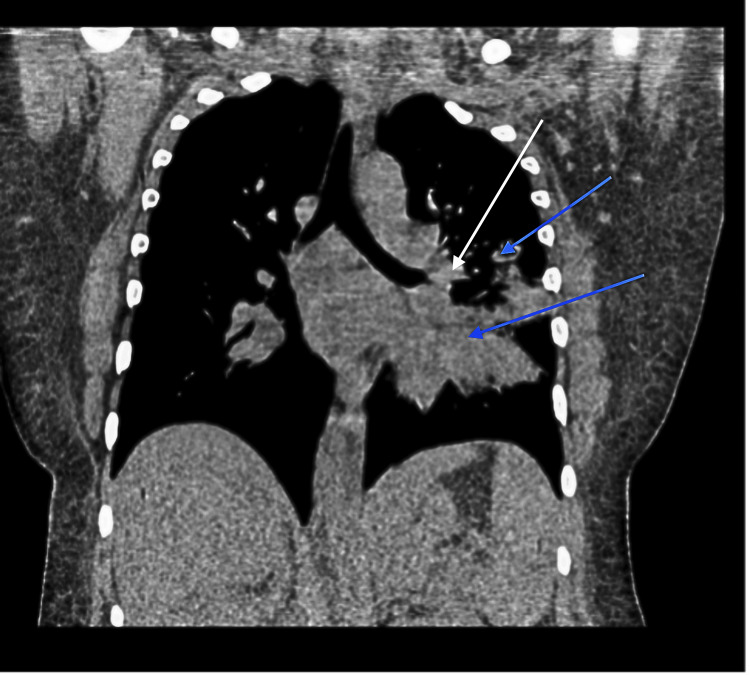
Coronal view of the CT scan showing multifocal pneumonia within the left upper and lower lobes (marked by blue arrows) and a left hilar opacity (marked by the white arrow)
